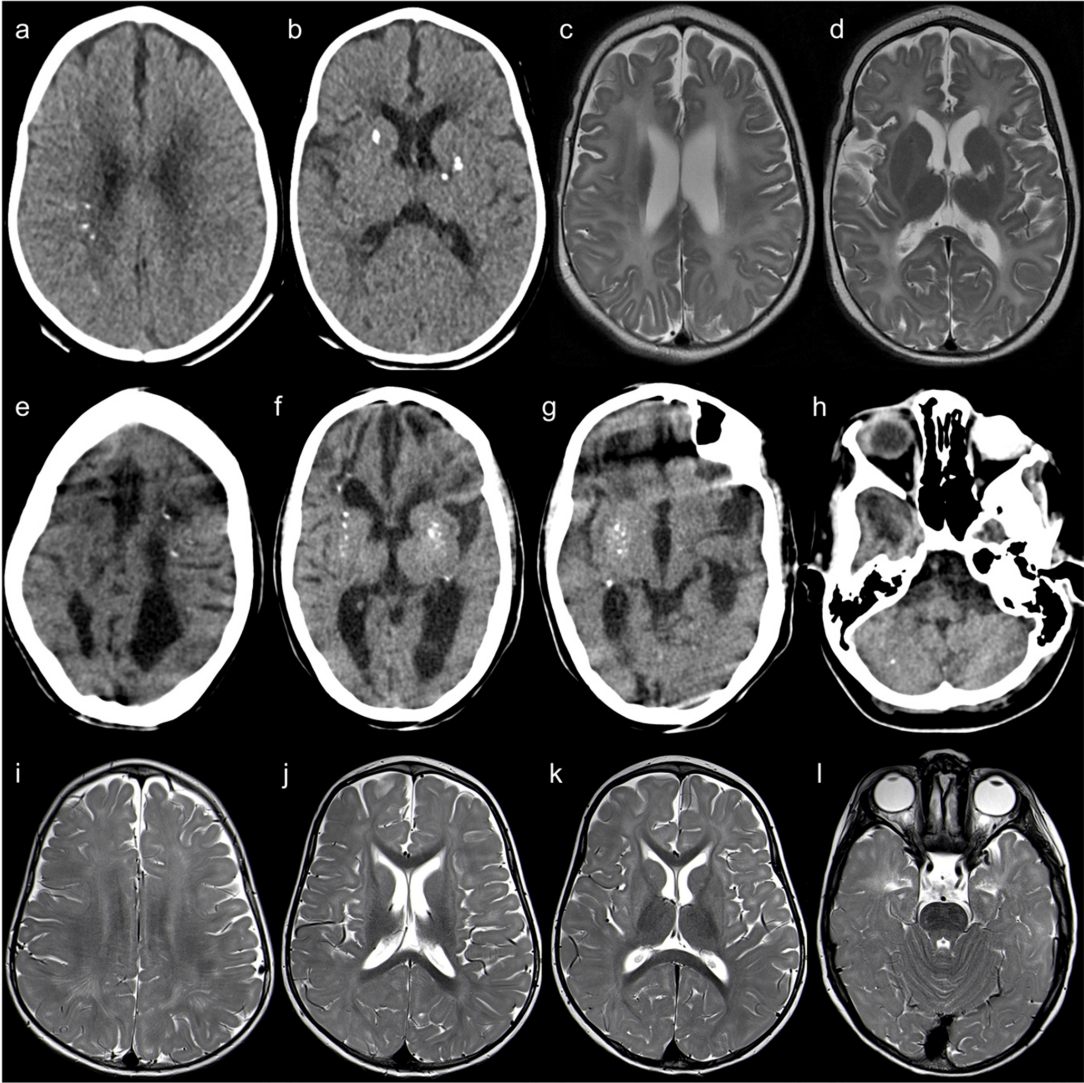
Different neuroradiologic patterns in Aicardi-Goutières syndrome. AGS type 5 (SAMHD1 mutations). An 8-month-old male patient with low birth weight, developmental delay, lower limb hypertonia, and axial hypotonia. Head CT scan (a and b) shows multiple spot-like calcifications in the basal ganglia, internal capsules, coronae radiatae, and right frontoparietal deep white matter. Brain MRI (c and d – axial T2) reveals predominantly periventricular leukoencephalopathy and a left basal ganglia sequelae, probably of vascular etiology. AGS type 5 (SAMHD1 mutations). A 9-year-old female patient with severe prenatal encephalopathy, epilepsy treated with vigabatrin, and moderate cognitive deficit. Head CT scan (e to h) shows millimetric spot-like calcifications in the basal ganglia, cerebral white matter, and right cerebellar hemisphere. There is also diffuse brain atrophy and leukoencephalopathy with multifocal hypodensities of the residual white matter and apparent frontal subcortical cystic areas. AGS type 6 (ADAR1 mutations). A 3-year-old patient with developmental regression, hypotonia, and macrocephaly. (i to l) Axial T2 images demonstrate bilateral striatal T2-hyperintense lesions and diffuse leukoencephalopathy
